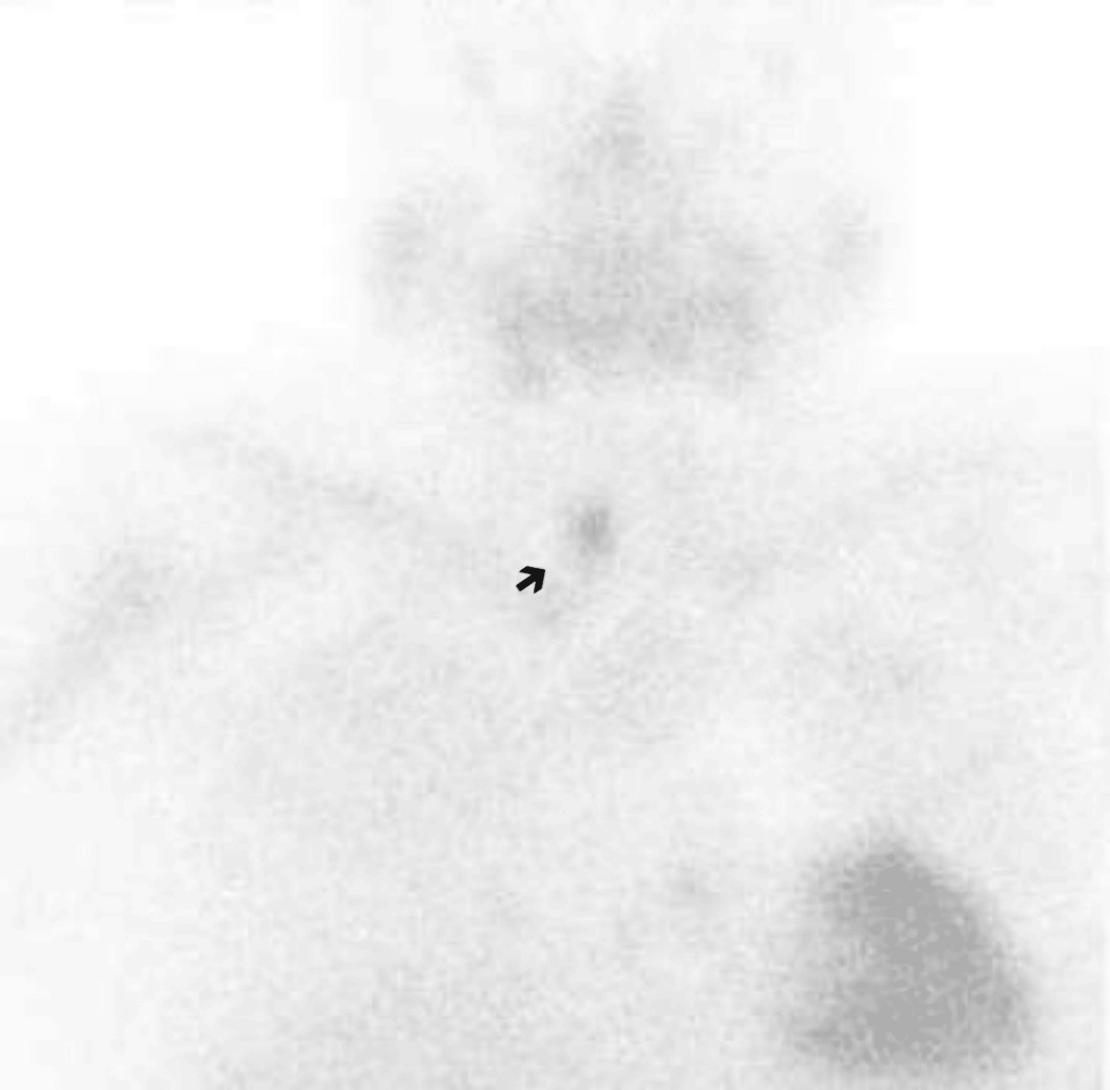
Parathyroid gland scintigraphy Arrow: right inferior parathyroid gland adenoma
